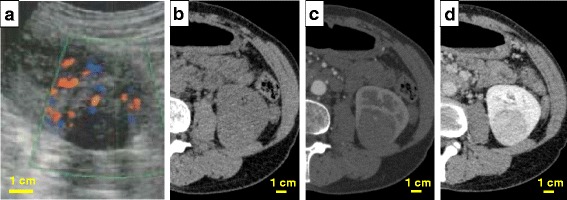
Clinical images of the left renal tumor. A mass with clear margin and mostly endophylic growth was detected in the lower and posterior part of the left kidney. a The tumor presented with a non-cystic mass of a heterogeneous nature in ultrasound. Color Doppler imaging showed hypervascularity in the tumor. b, c, d) Dynamic computed tomography of the left renal tumor. b Image before administration of the contrast medium. c Early phase of enhanced computed tomography. A blood vessel was visualized in the tumor. d The tumor showed earlier washout of enhancement than the adjacent normal renal parenchyma
